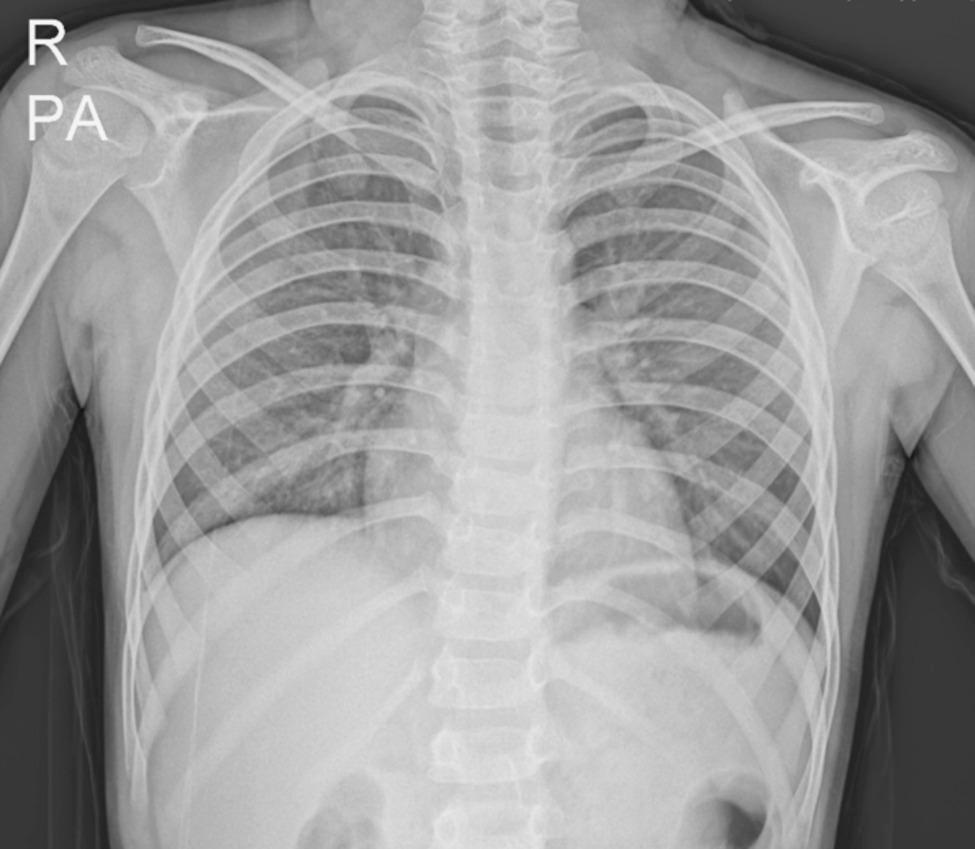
X-ray chest PA view. No significant abnormality detected
